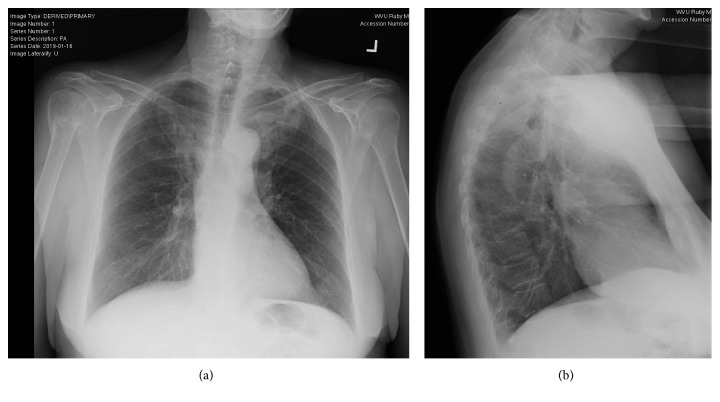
PA and lateral chest x-rays performed on 1/16/2019. (a) PA view with asymmetry at the left apex, which could relate to osseous structures, with questionable expansion of the proximal left first anterior rib, and (b) lateral view with questionable expansion of the inferior sternum.
